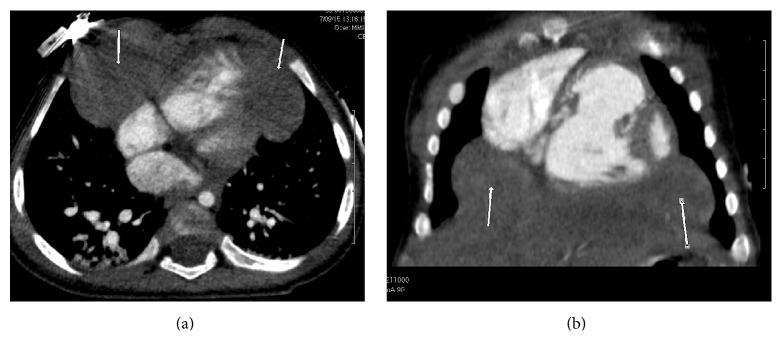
Axial (a) and coronal (b) images from a cardiac CTA of the chest show focal, bilateral anteromedial herniation of liver (arrows).
